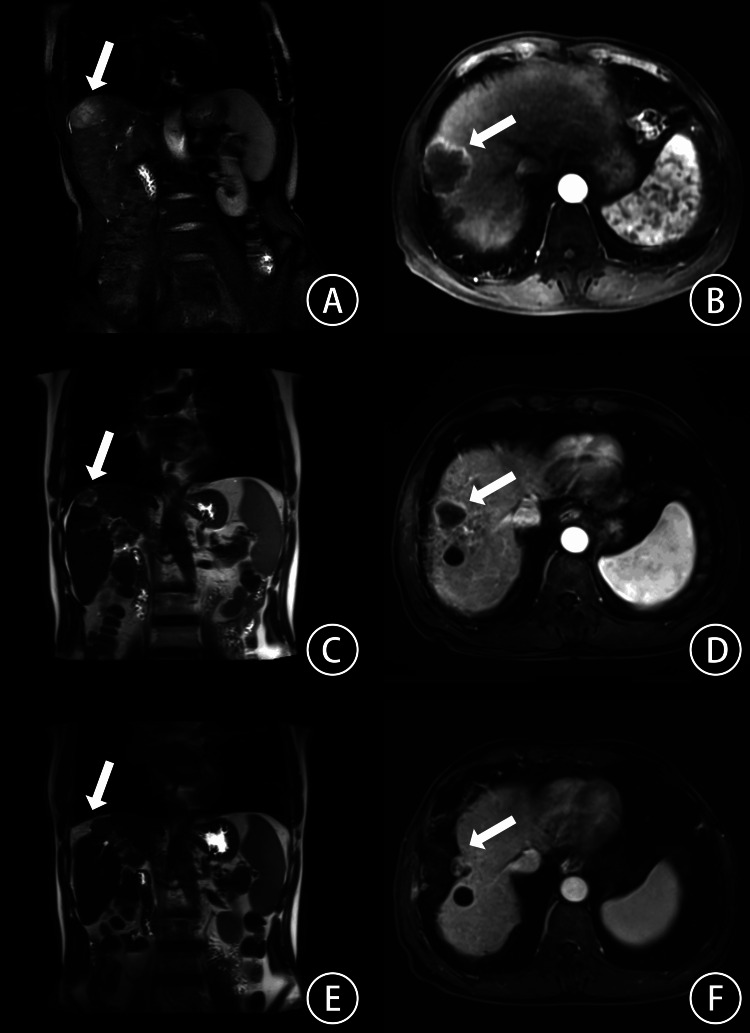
The MRI scan before and after SBRT A, C, and E. Coronal plane scanning; B, D, and F. Cross-sectional scanning. A and B. MRI scan before SBRT, C and D. MRI scan two months after SBRT, and E and F. MRI scan 17 months after SBRT. MRI, magnetic resonance imaging; SBRT, stereotactic body radiation therapy.
